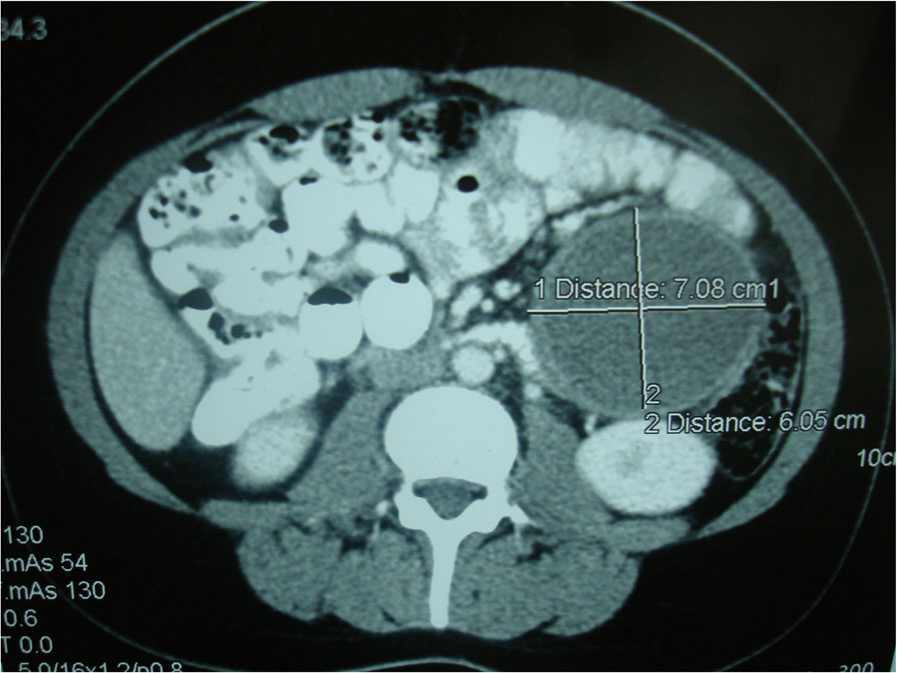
Computed tomography showed a well-defined hypodense cystic 7.08 × 6.05 cm chylous cyst with mild enhancement
